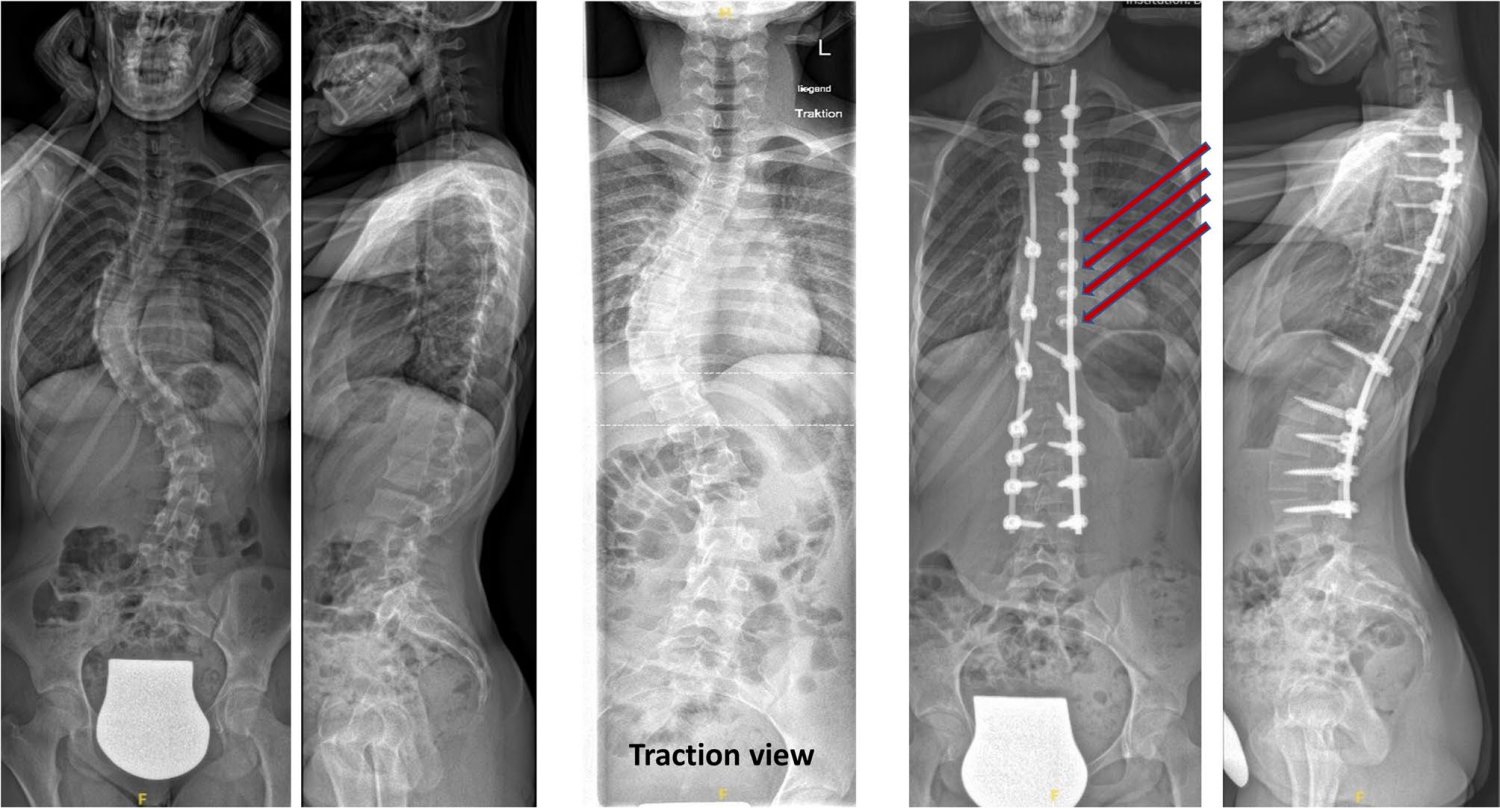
Pre- and postoperative A.P. and lateral radiographs of a 14-year-old female patient with idiopathic adolescent scoliosis, treated with posterior spinal fusion and the additional use of four sublaminar bands applied at the apex of the main curve
